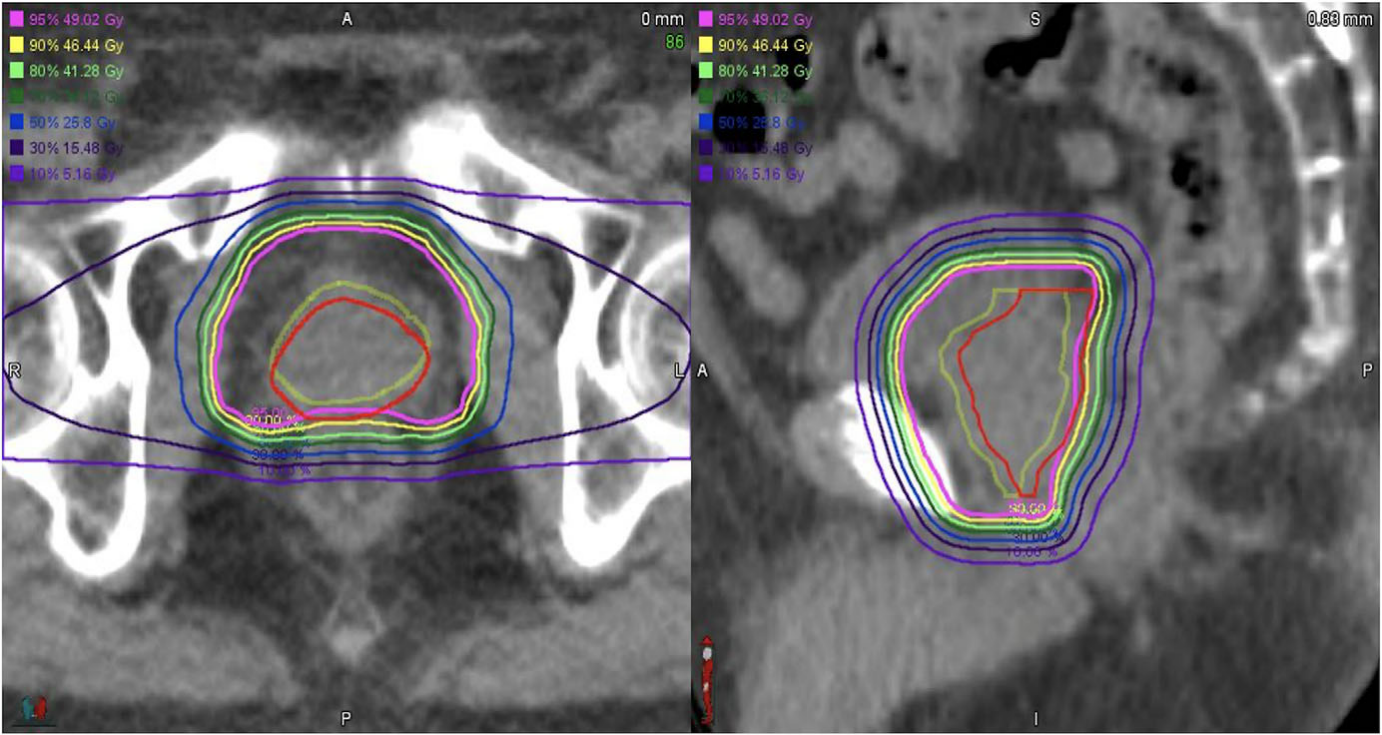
Representative reproduction of the dose distribution. The yellow contoured line shows the clinical target volume (CTV) on planning computed tomography (CT), and the red line denotes the CTV on in‐room CT.
